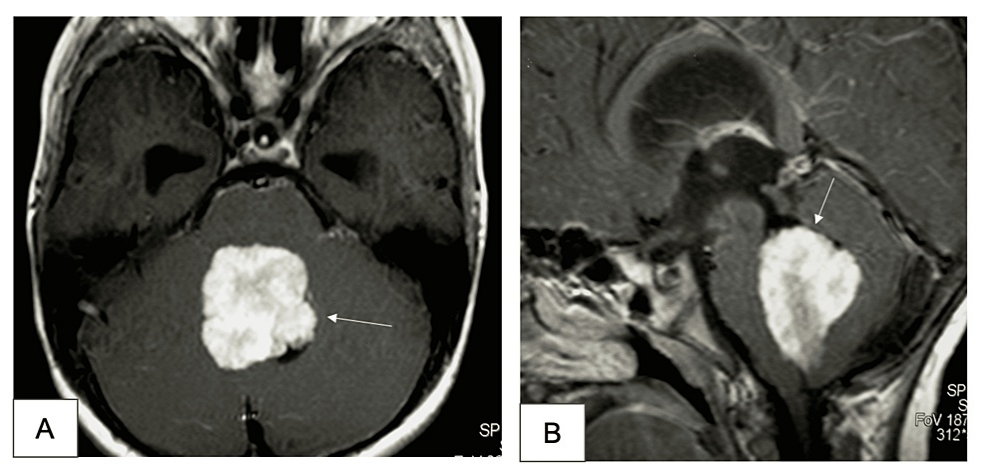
Axial (A) and sagittal (B) MRI images show a fourth ventricular tumor that lead to obstructive hydrocephalus MRI = magnetic resonance imaging
